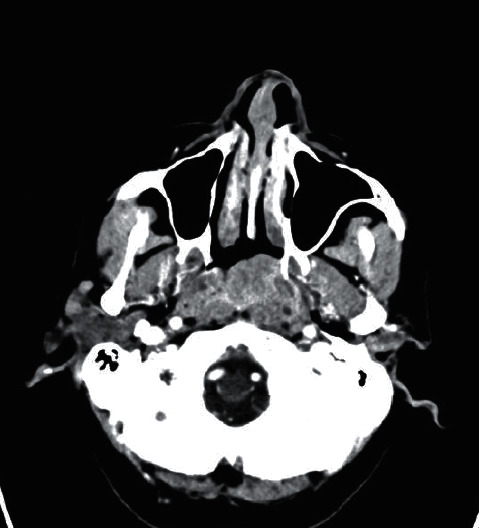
Axial CT imaging of the head showing the nasopharyngeal mass.
